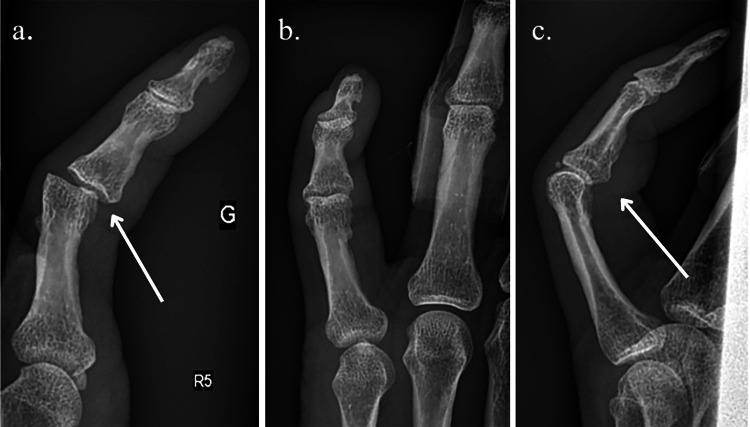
X-rays before and after reduction in the emergency department. (a) Initial X-ray (white arrow: ulnar subluxation of the PIP joint). (b,c) X-rays after reduction ((c) white arrow: flexum of the PIP). PIP, proximal interphalangeal
